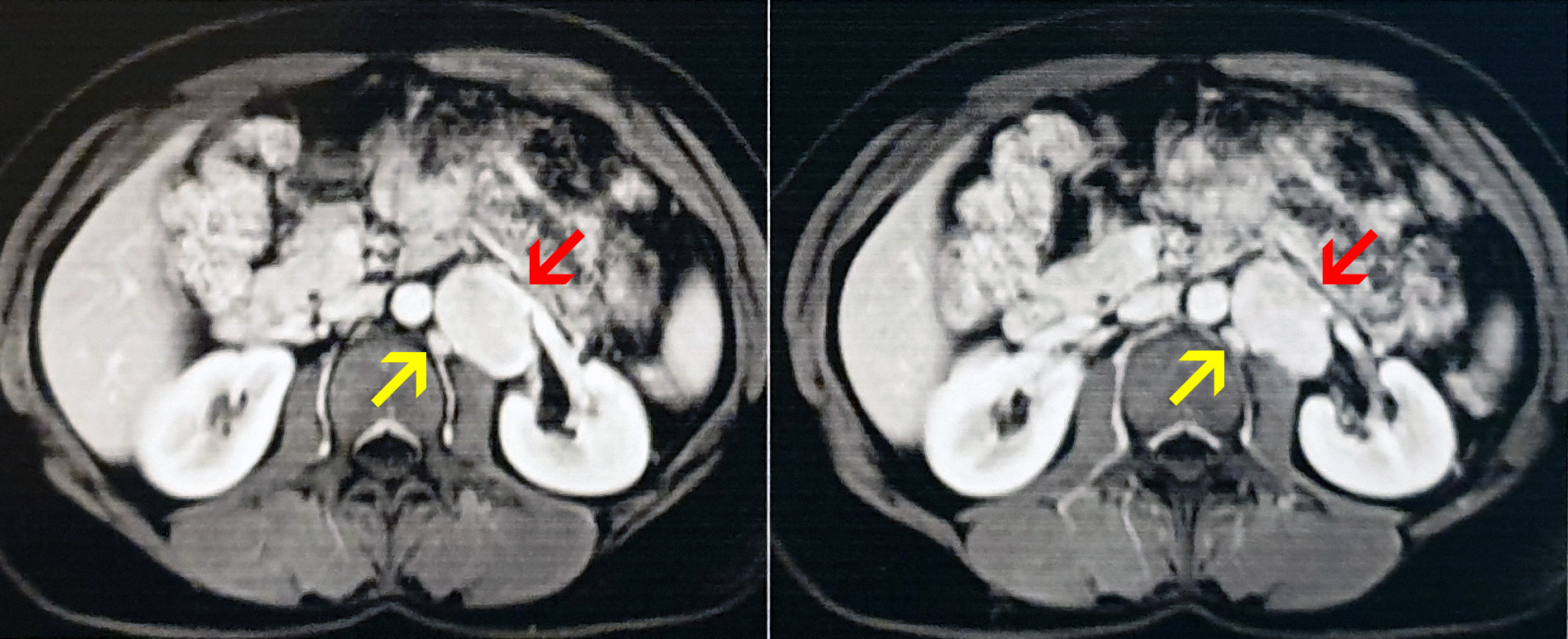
Abdominal CT scan with intravenous contrast shows iso‐dense mass (red arrow) and para‐aortic lymph node (yellow arrow).
